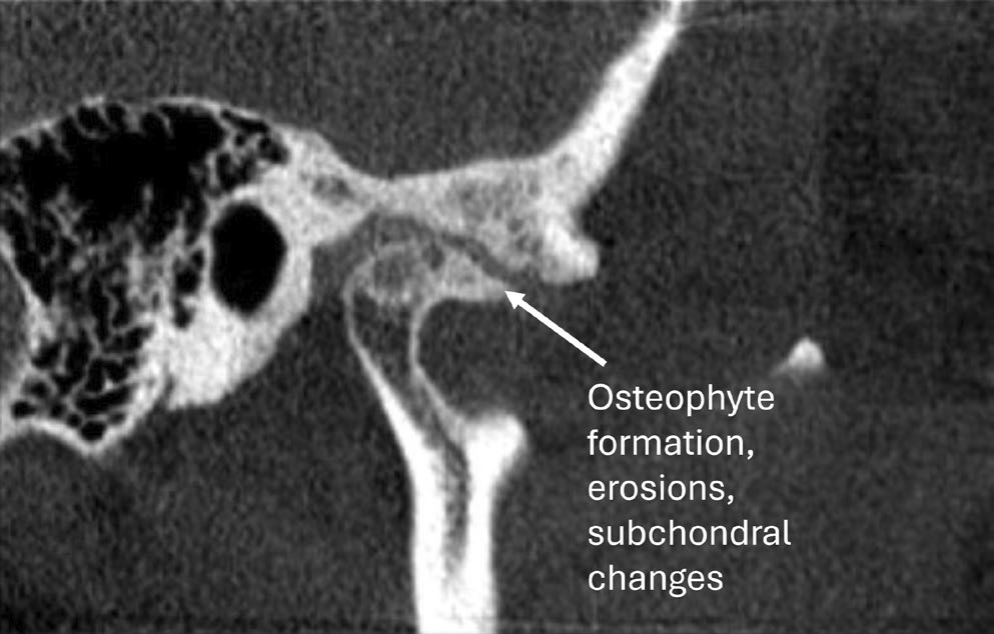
Sagittal CBCT view of right TMJ with osteophyte formation. Sagittal view showing osteophyte formation in the right condyle, along with erosion and subchondral changes in both the condyle and articular eminence.
